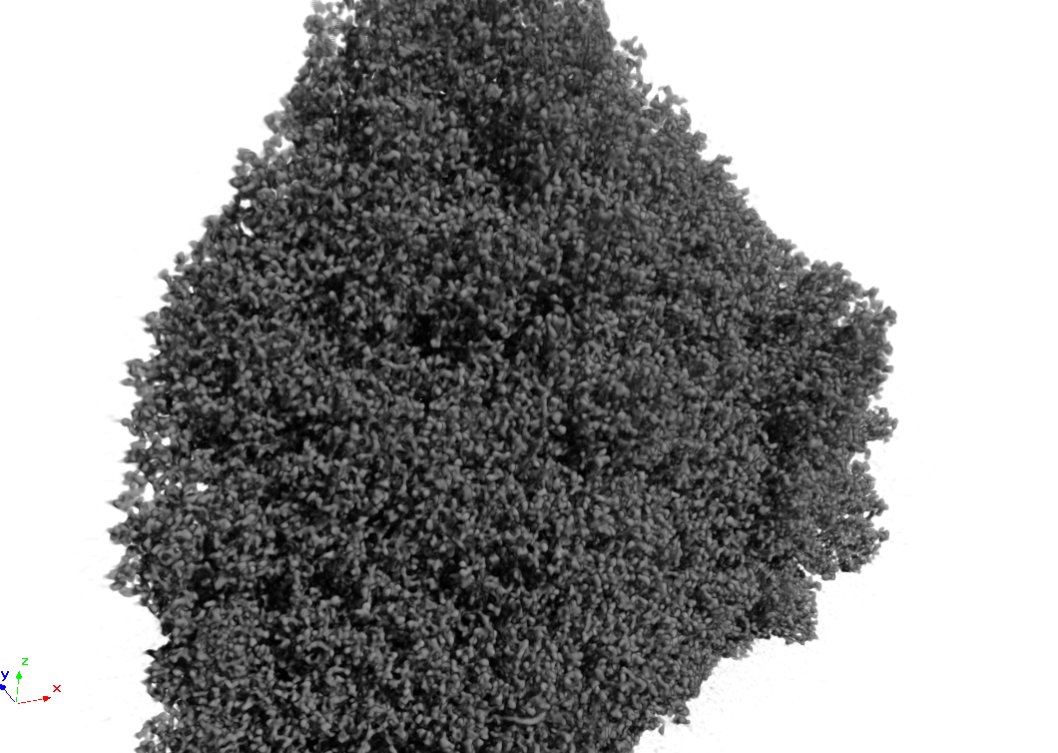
X-ray tomography 3D-reconstruction image of MonoBor [133]. Unpublished image from the authors.
